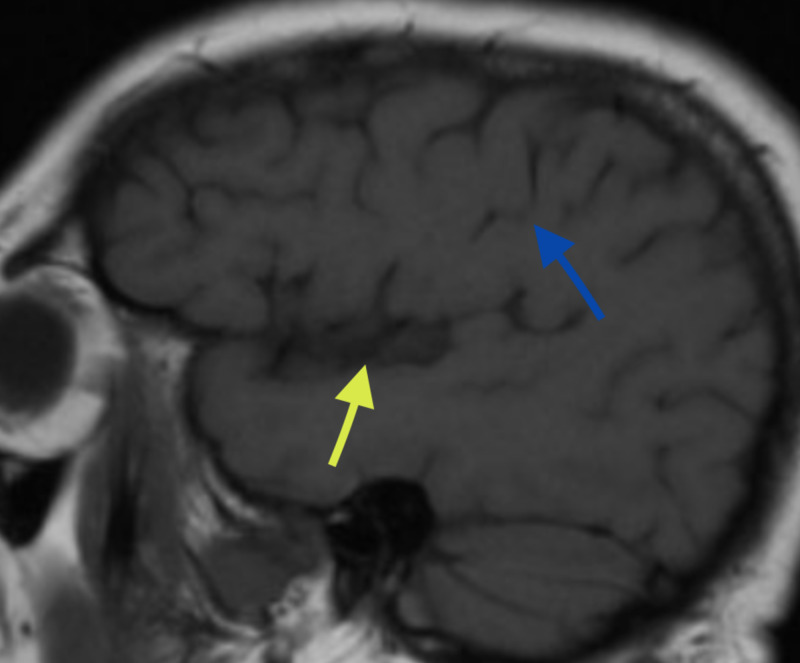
T1-weighted coronal MRI The image shows insula (yellow arrow) hook of omega sulcus on coronal view (blue arrow) MRI: magnetic resonance imaging
